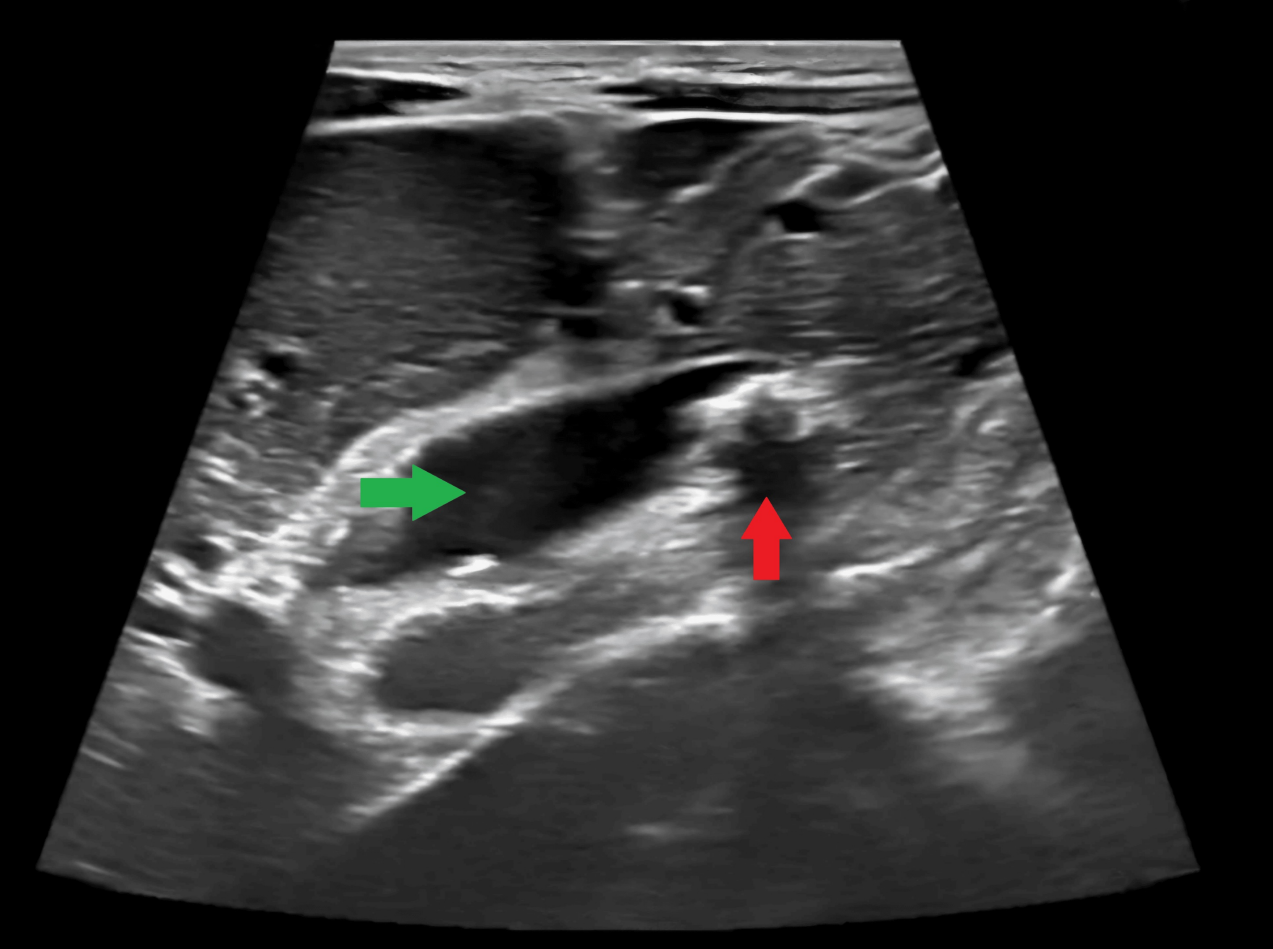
Ultrasound of abdomen showing calculus at the distal common bile duct causing proximal bile duct dilatation. The green arrow shows the common bile duct, and the red arrow shows the calculi
